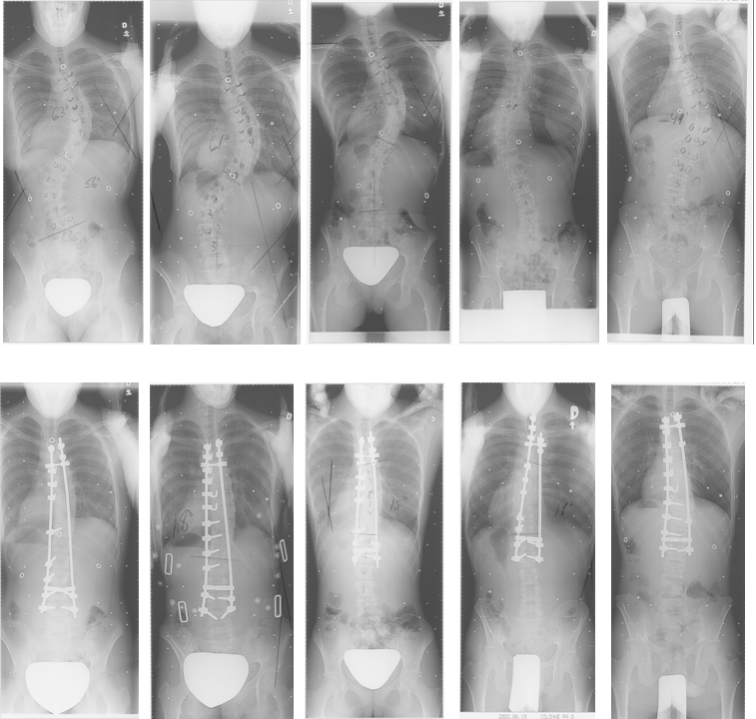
Pre- and post-operative radiographs, presented respectively on top and bottom row.
